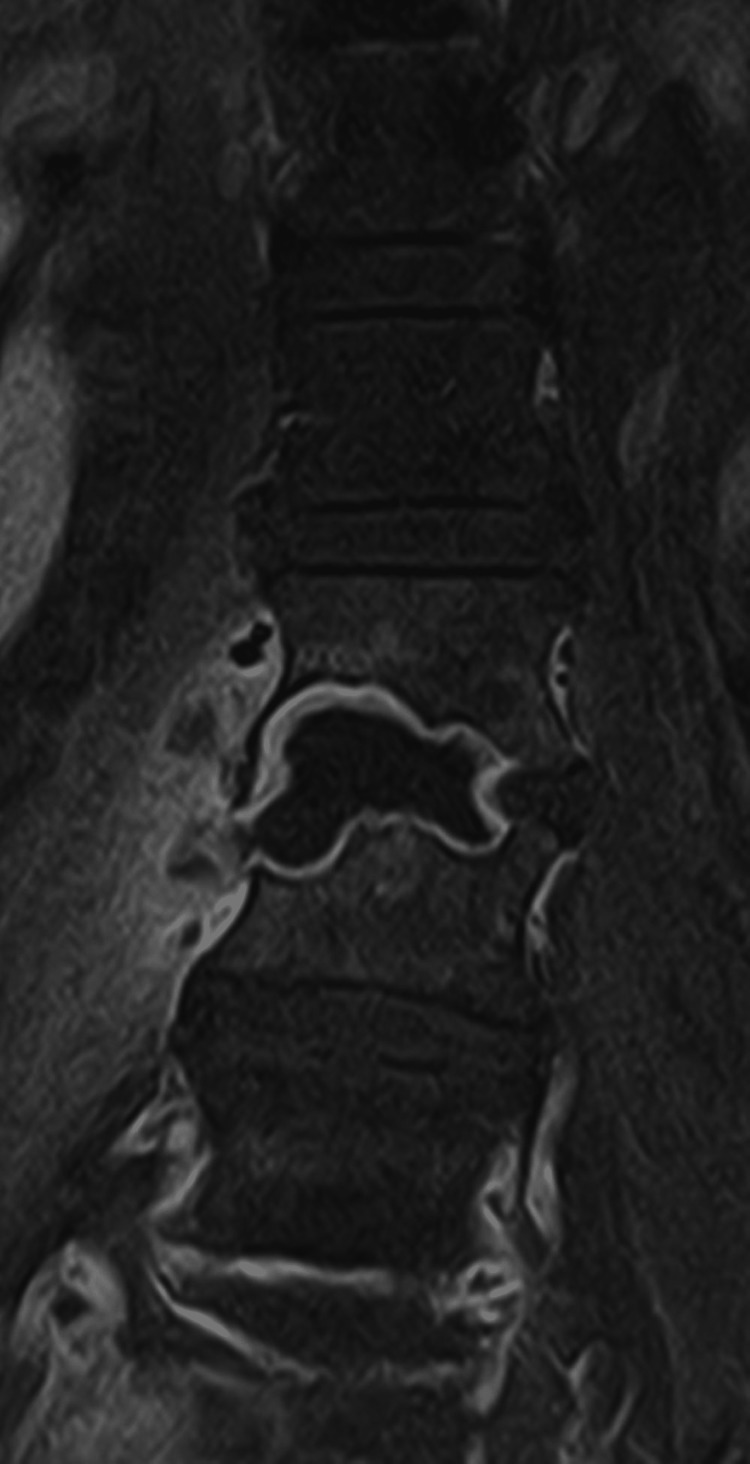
MRI six weeks after the start of the treatment (coronal view).
